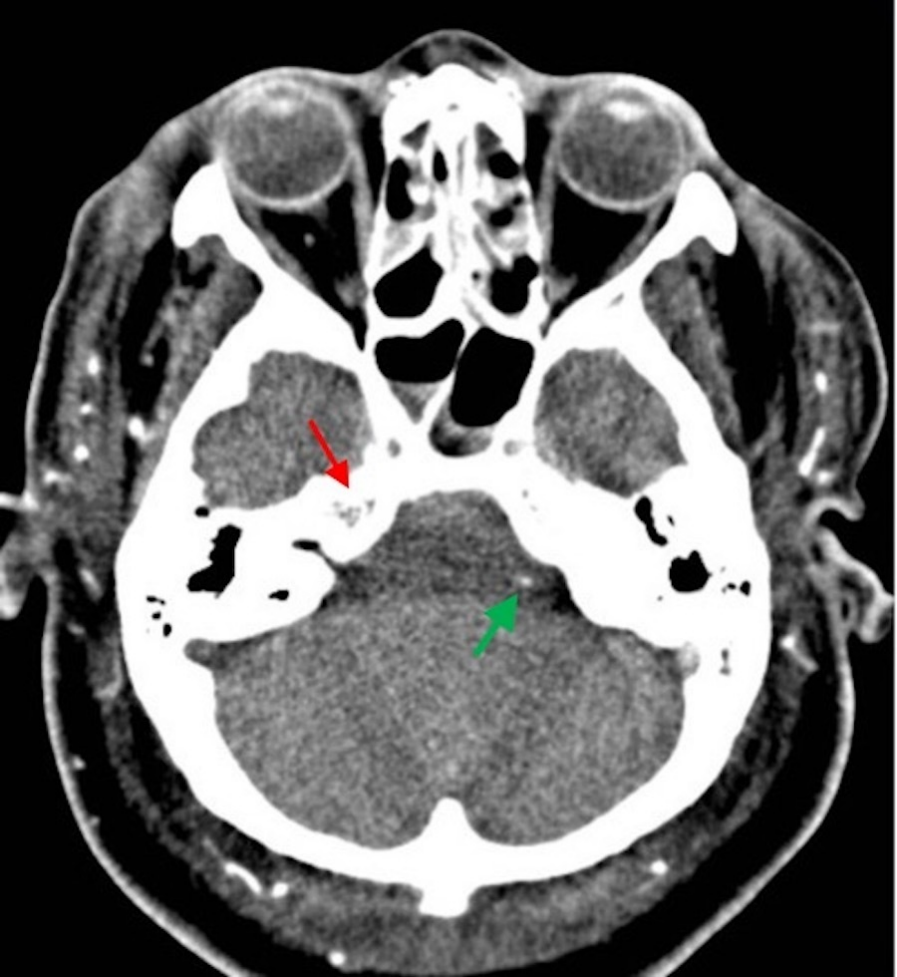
Axial CT angiography of intracranial arteries. Image shows the flow in the petrous segment of internal carotid (red arrow) and proximal intradural vertebral arteries (green arrow) but not thereafter.
